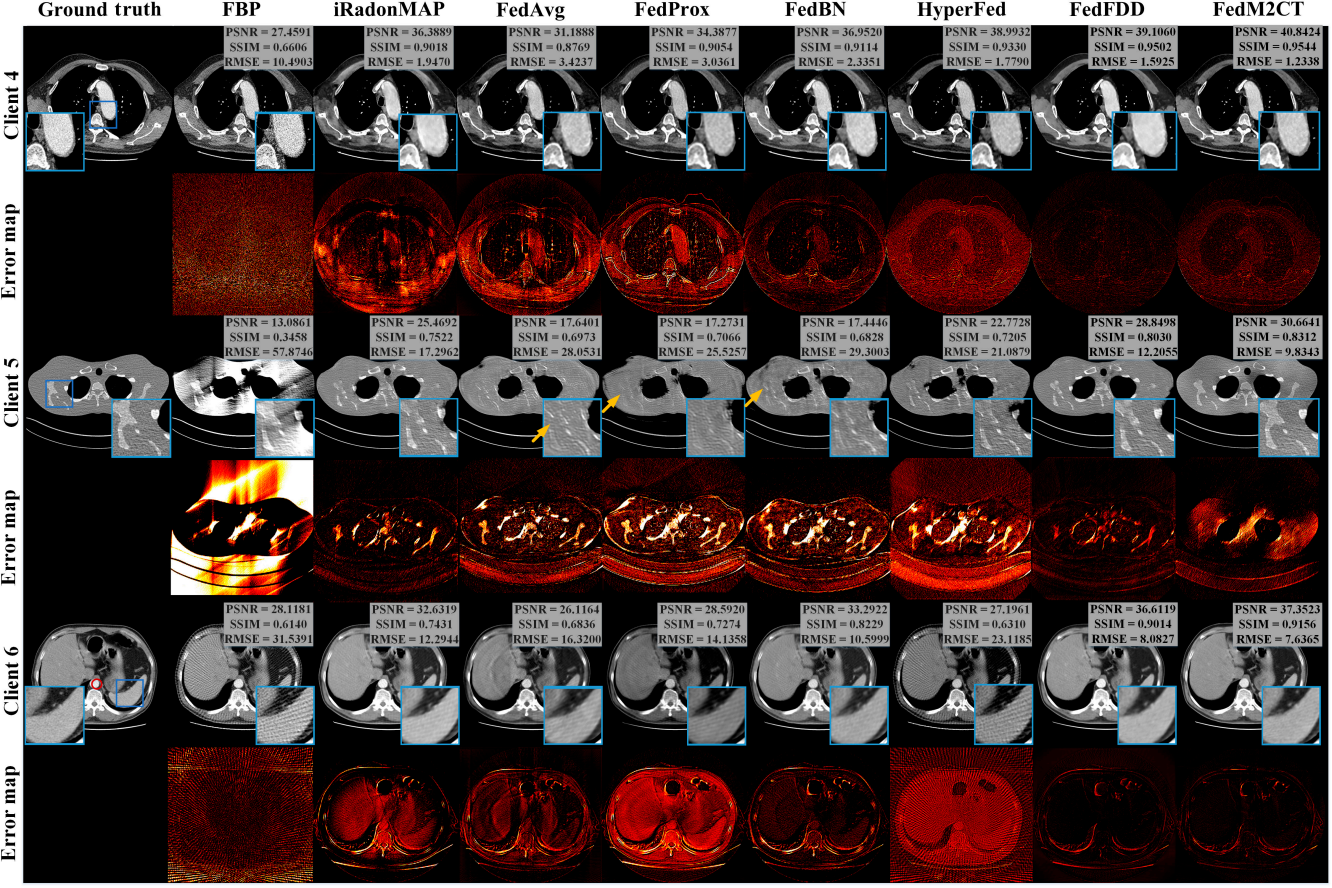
Visual comparisons of the representative images reconstructed by different methods from 3 clients in situation 2. Zoomed-in ROIs indicated by the red boxes are shown on the corresponding images. The quantitative measurements are also listed. The display windows of client 4 and client 6 are [−160,240] HU for the CT images and the zoomed-in ROIs, and the display windows of client 5 are [−400,400] HU for the CT images and the zoomed-in ROIs.
